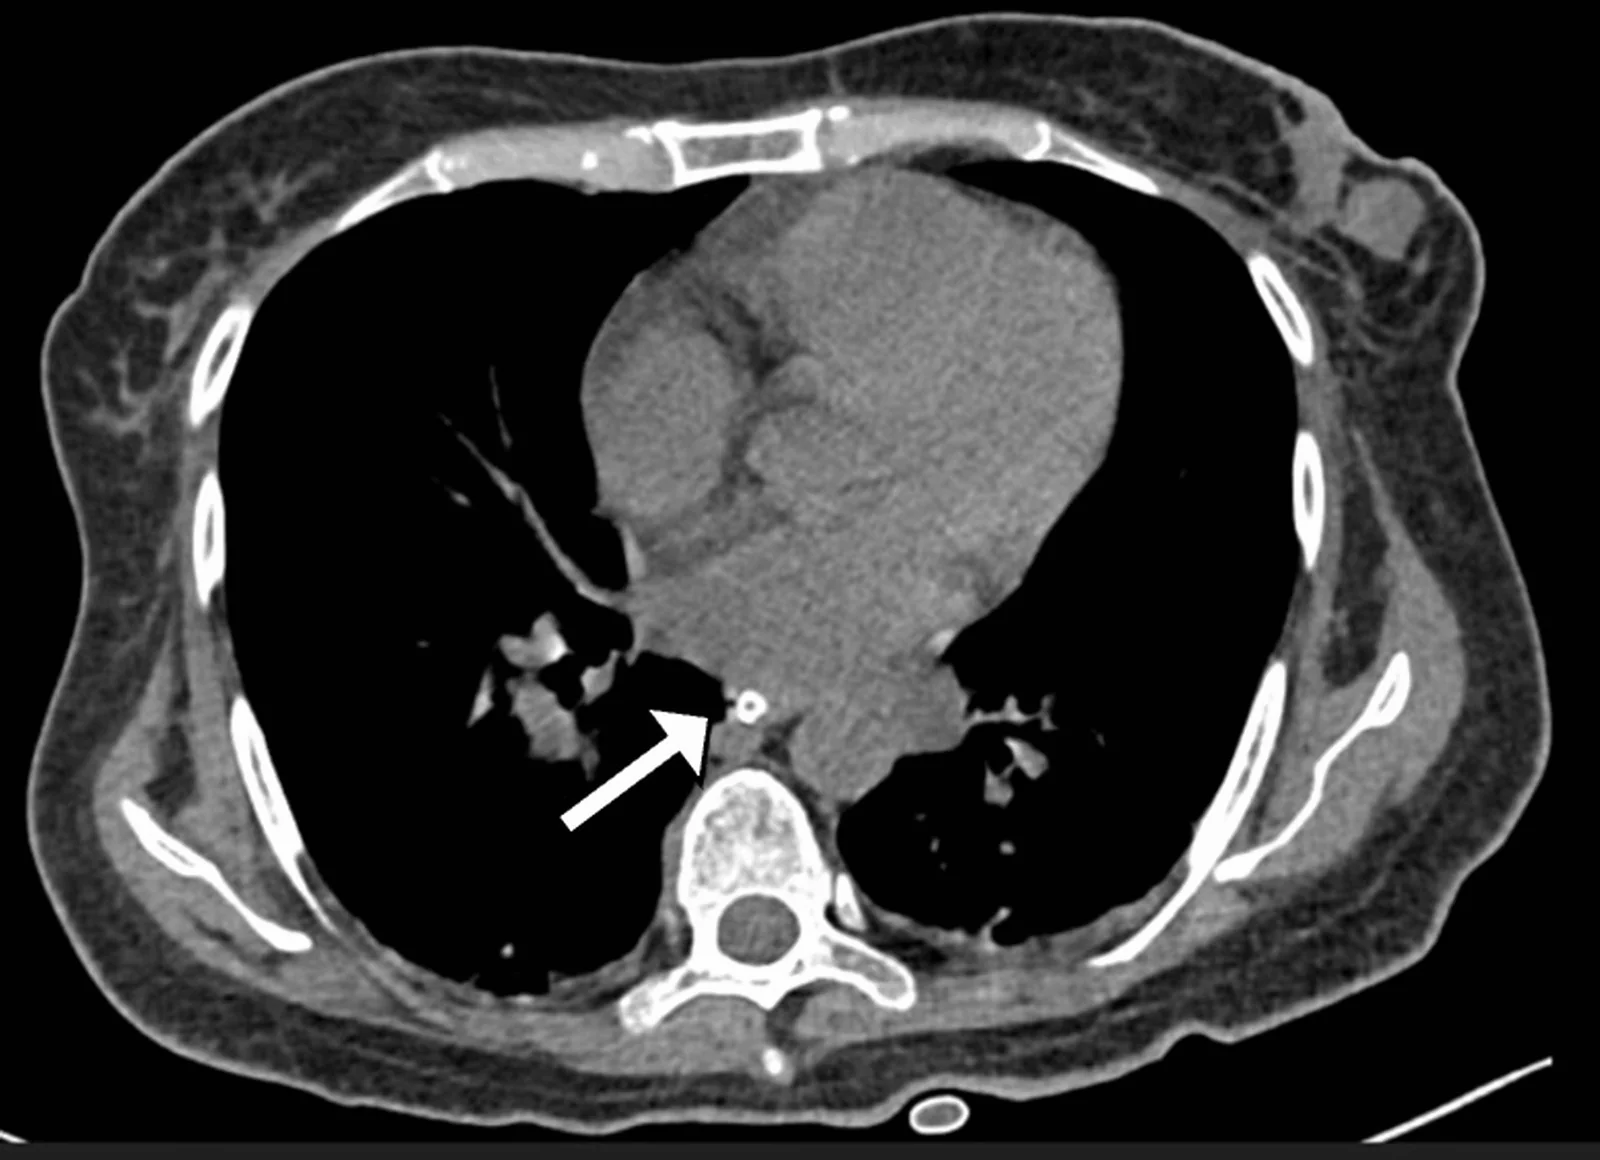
Axial computed tomography image of the thorax. The white arrow highlights the radiopaque nasogastric tube along the expected esophageal tract. No clear evidence of esophageal disruption, mediastinal collection, or pneumomediastinum is observed.
